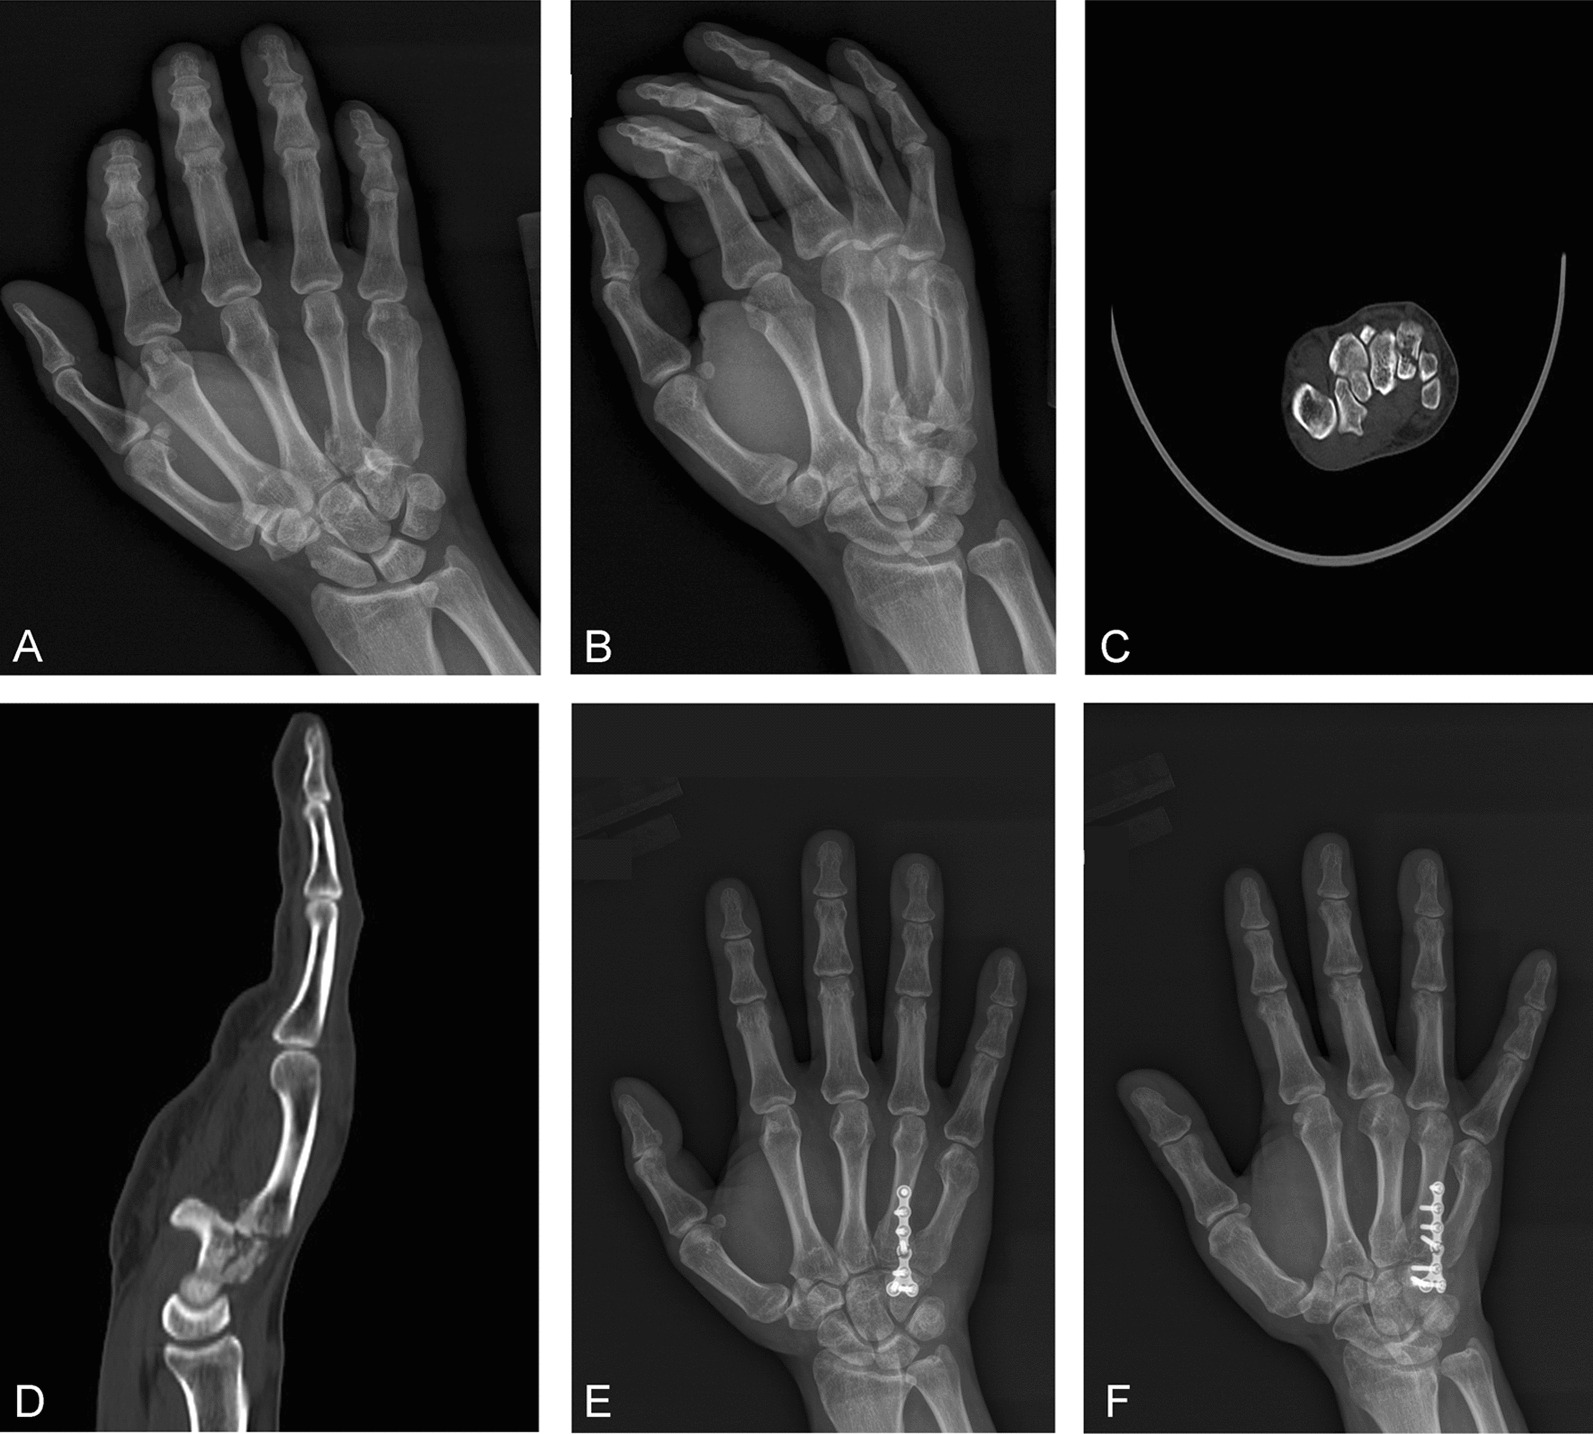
A, B The preoperative anteroposterior oblique films of the affected hand indicate that the fracture of the base of the fourth metacarpal bone is complicated with dislocation. C, D Preoperative computed tomography images of the affected hand indicate that there is dorsal dislocation of the fracture of the base of the fourth metacarpal bone and a hamate bone fracture. E, F Three months postoperatively, the posteroanterior and lateral oblique views on radiograph show that the transarticular steel plate is broken
